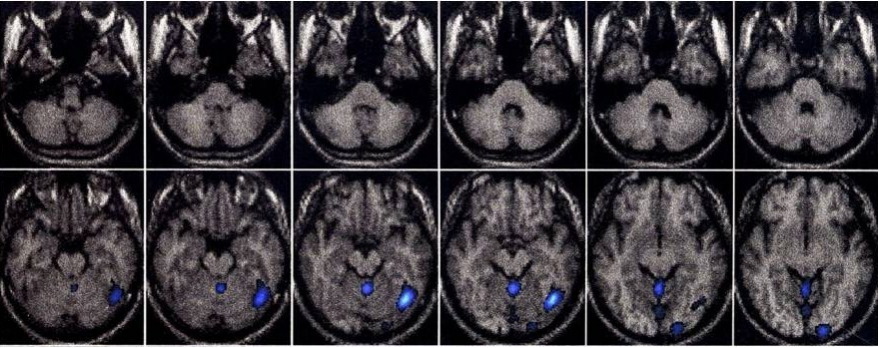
SPECT-eZIS. SPECT-eZIS revealed reduced cerebellar blood flow, which was left hemisphere dominant.
